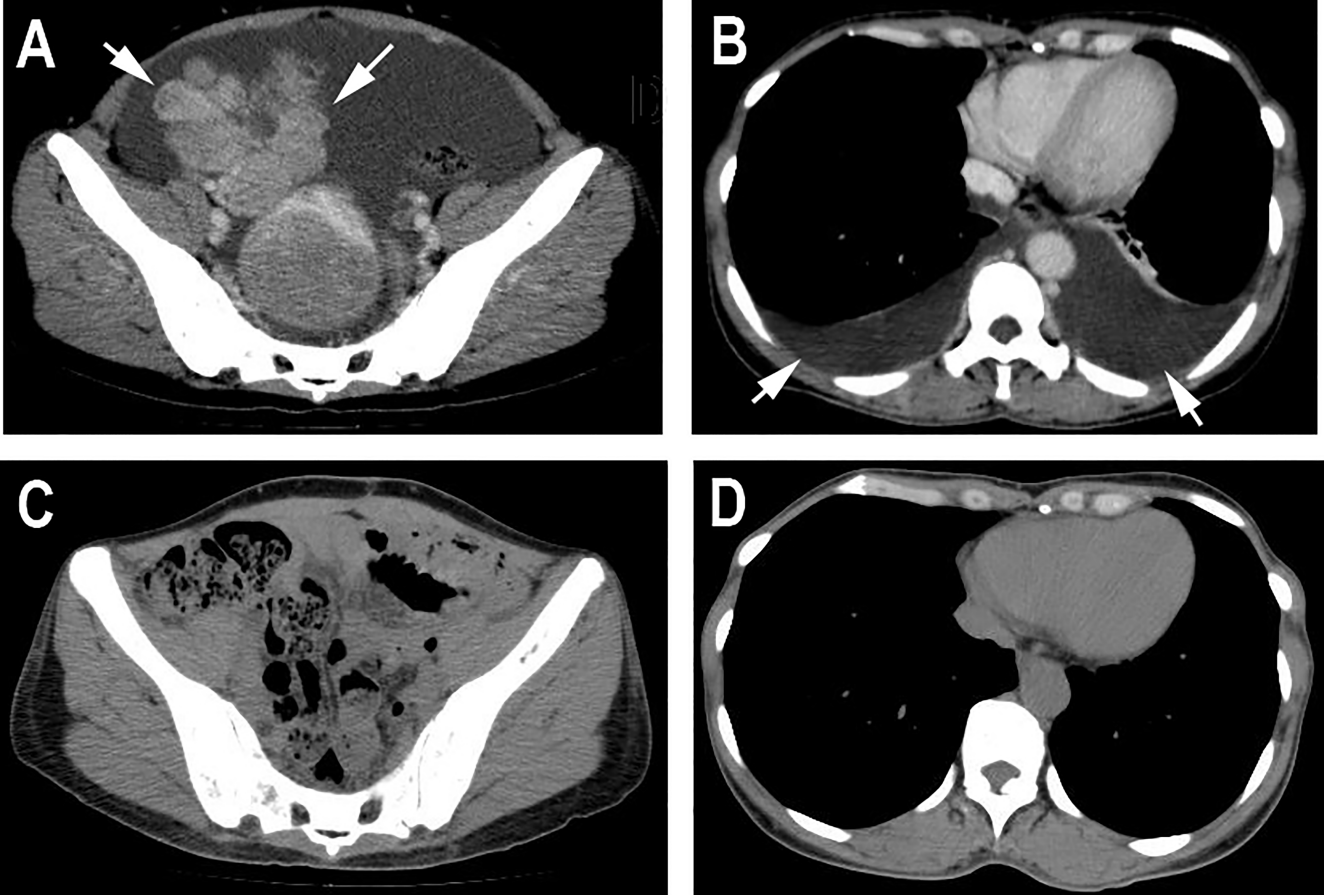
Computed tomography. (A) Pelvic CT before the surgery showed a huge mass in the middle and lower abdomen accompanied by ascites. (B) Chest CT before the surgery showed bilateral pleural effusion and partial dilatation of the lower lobe of both lungs. (C, D) CT after the surgery showed that the huge masses in the pelvis and the bilateral pleural effusion had disappeared.
